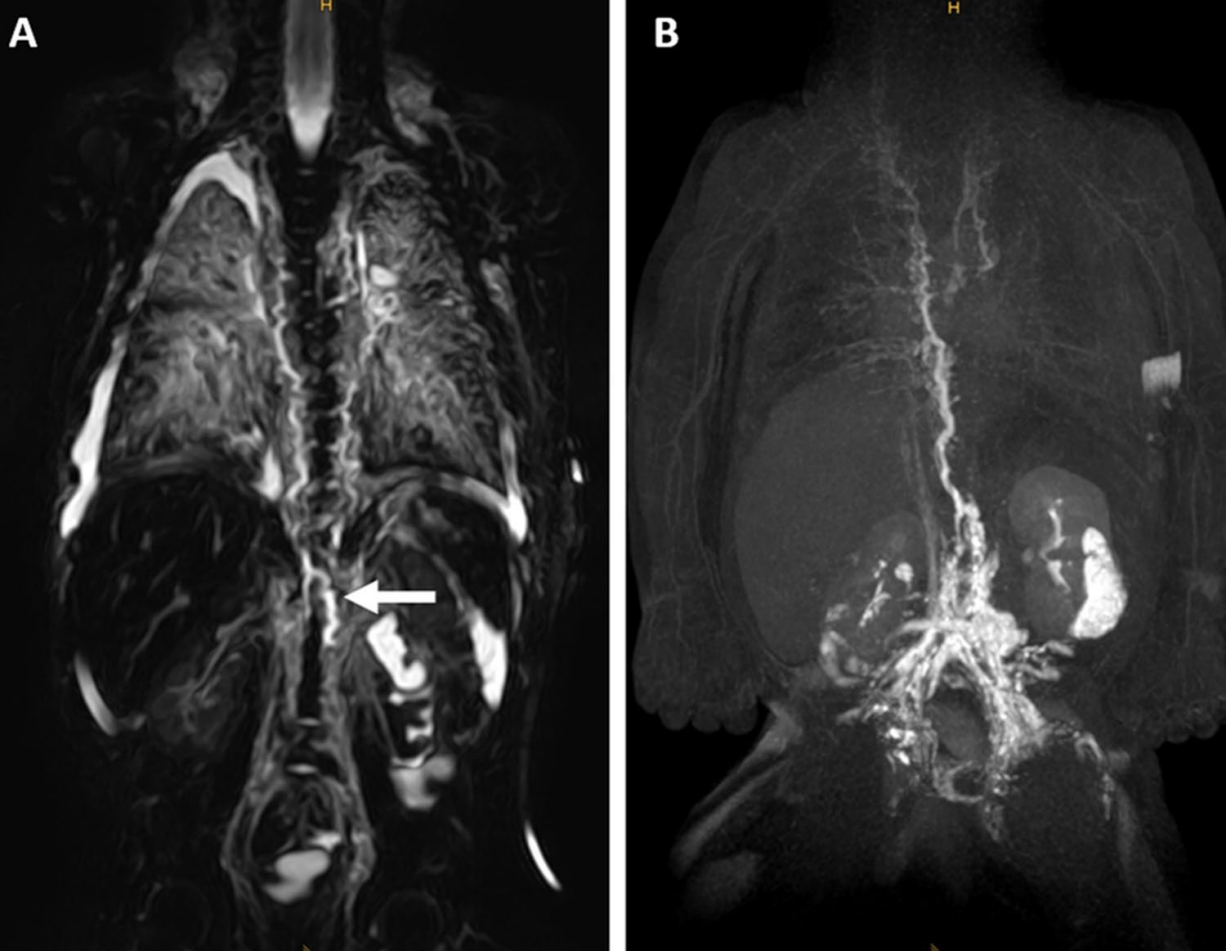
Two-month-old boy with Noonan syndrome-associated bilateral chylothorax and pulmonary lymphangiectasia. (A) Coronal, T2-weighted, non-contrast imaging demonstrates the right-sided course of the thoracic duct from the cisterna chyli (arrow) into the middle part of the thorax where it can no longer be distinguished from surrounding edema. Observe also the extensive pulmonary abnormalities and bilateral pleural effusions. (B) Coronal maximum-intensity projection (MIP) of a contrast-enhanced MR-lymphangiogram demonstrating dilated retroperitoneal lymphatics, the dilated cisterna chyli and thoracic duct in the lower and middle part of the thorax. The thoracic duct runs entirely on the right side and is only rudimentary in the upper part of the thorax. There is also cross-flow through mediastinal lymphatics to the left venous junction. There is only faint enhancement of cervical lymphatics without a discernable TD-venous junction of the thoracic duct. Observe also faint reflux into bilateral intercostal lymphatics and peribronchial lymphatics on the right.
